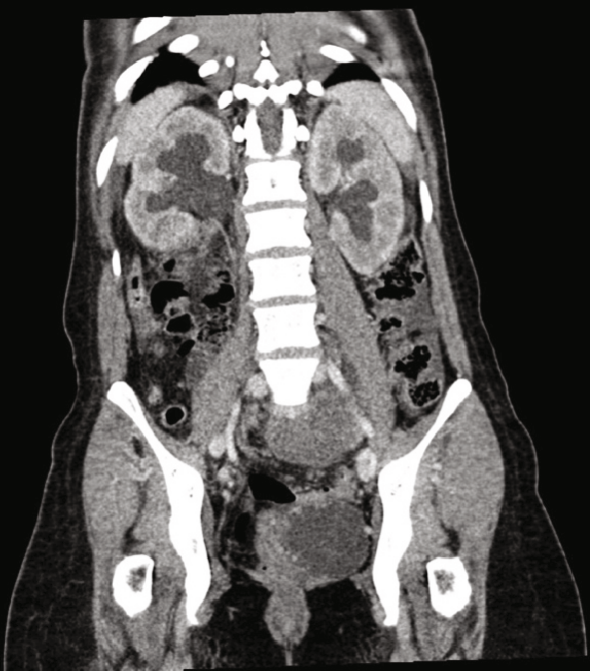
Preoperative contrast-enhanced CT demonstrated bilateral hydronephrosis, secondary to compression from the large adnexal mass.
